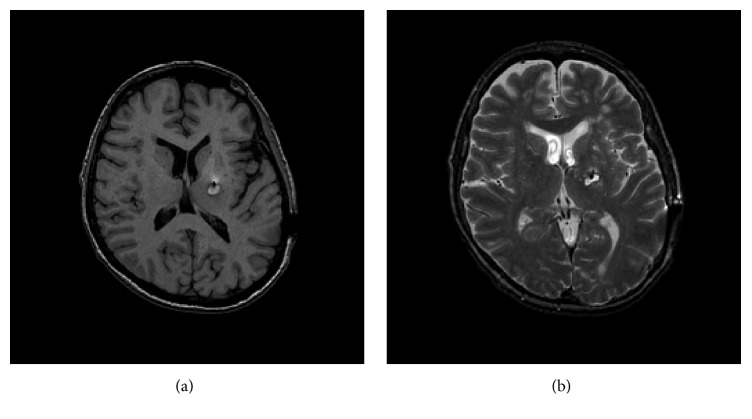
(a) An MRI brain confirmed a small resolving hematoma at the tip of the lead. (b) An MRI brain confirmed a small resolving hematoma at the tip of the lead.
